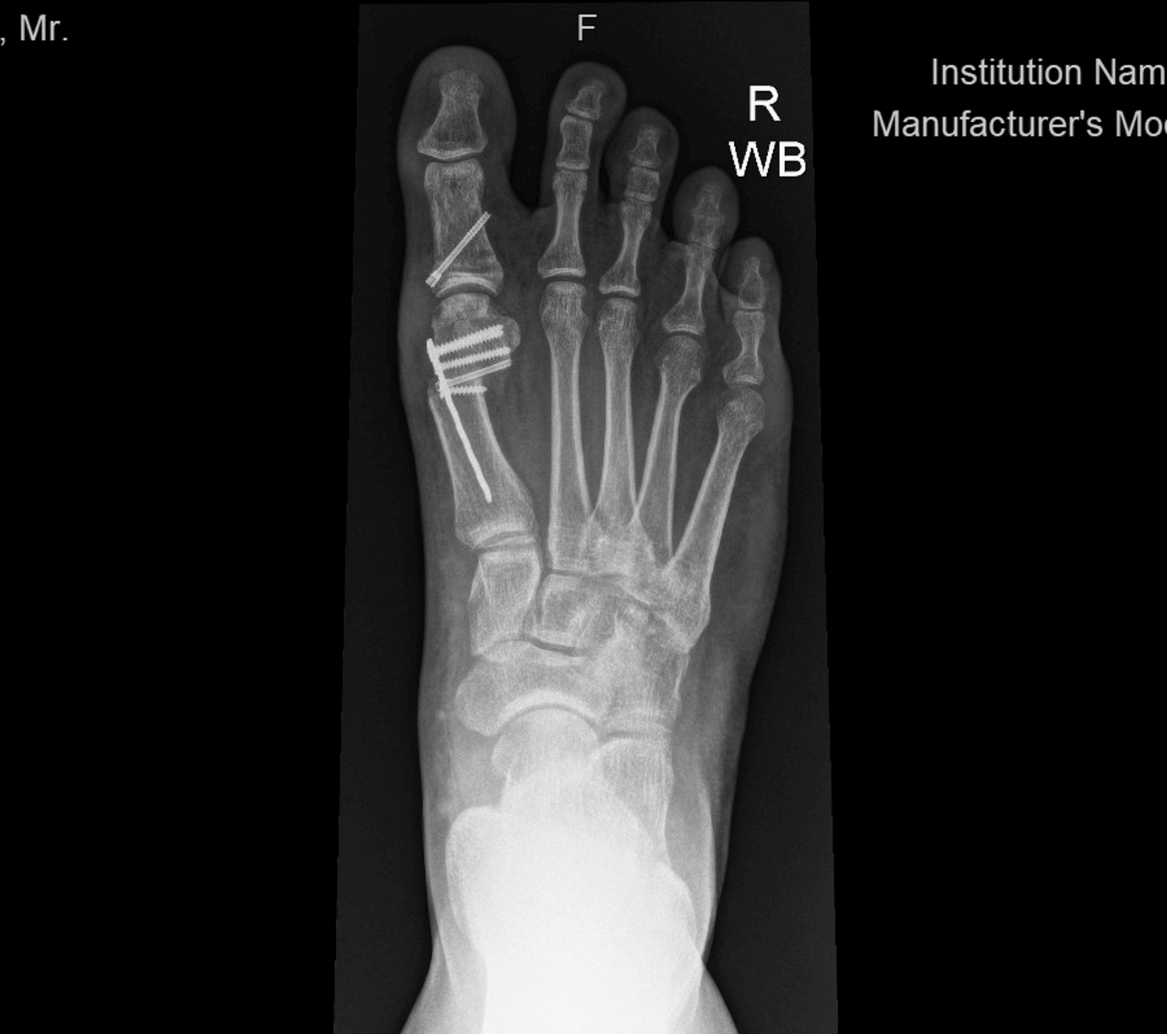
Post-operative weightbearing AP foot radiograph showing the intramedullary plate inserted AP: anterior-posterior
